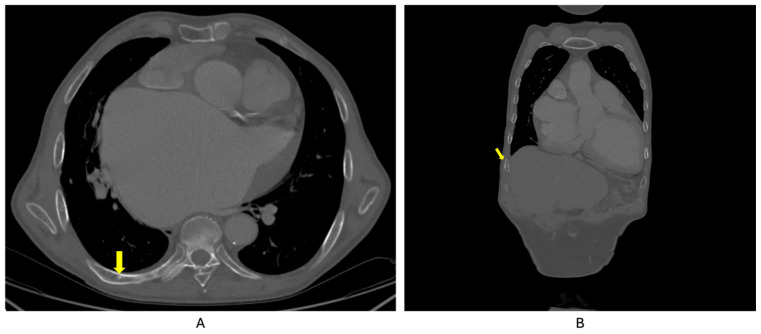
CT showing (A). Pagetic lesions (yellow arrows) of the 7th rib in the axial plane; (B). Pagetic lesion of the 7th rib in the coronal plane.
